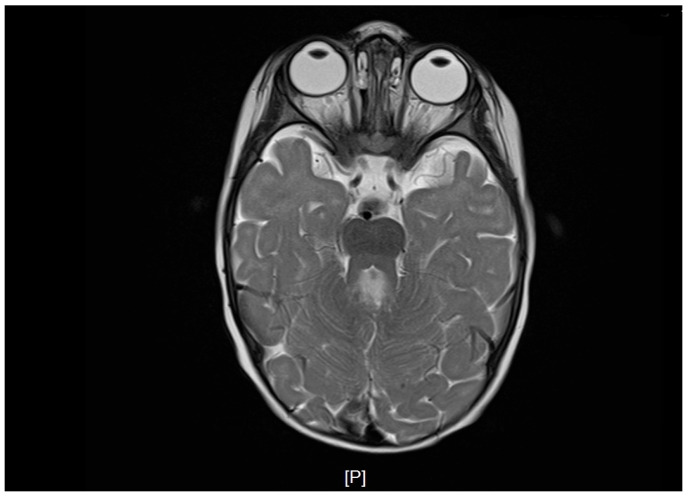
Brain MRI demonstrating the molar tooth sign characteristic of Joubert syndrome. Axial T2-weighted image shows vermian agenesis and elongated superior cerebellar peduncles.
